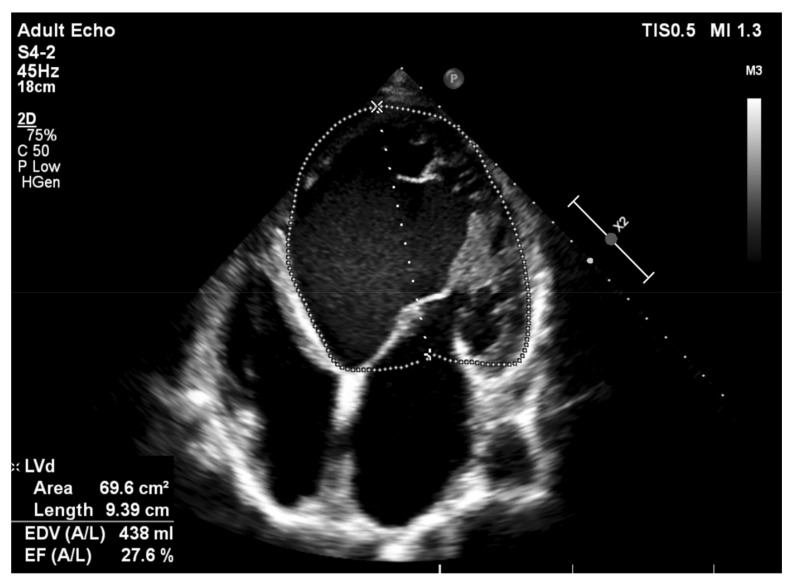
Transthoracic echocardiography—apical 4-chamber view: severe left ventricular dilation with markedly decreased ejection fraction in a 47-year-old subject with a history of long-term alcohol abuse (authors’ personal archive).
